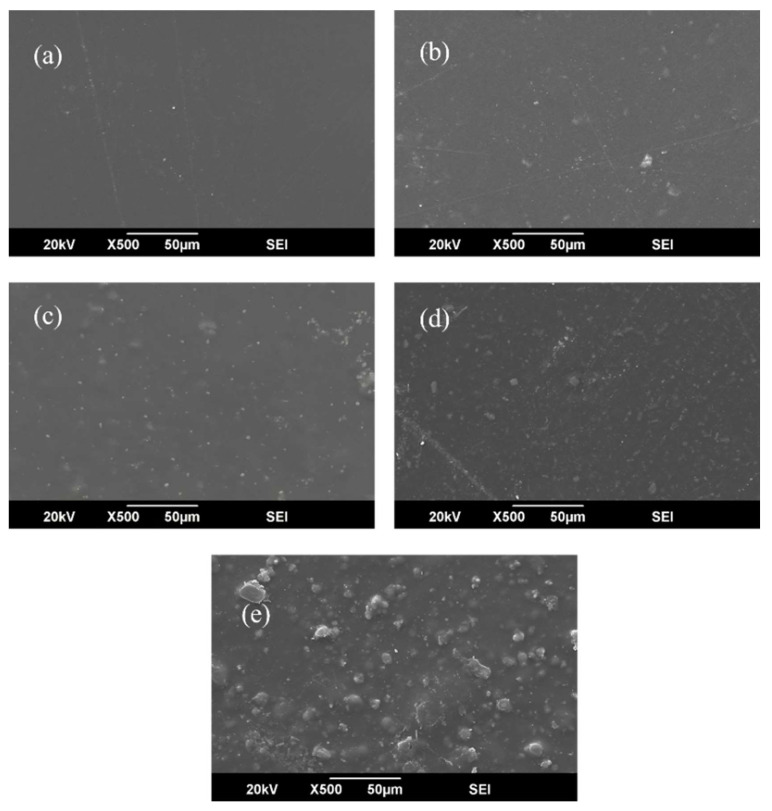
Surface morphology of membranes: (a) pristine CTA, (b) CTA/Cu-BTC-5 wt.%, (c) CTA/Cu-BTC-10 wt.%, (d) CTA/Ni-Cu-BTC-10 wt.%, and (e) CTA/Cu-BTC-15 wt.%.
